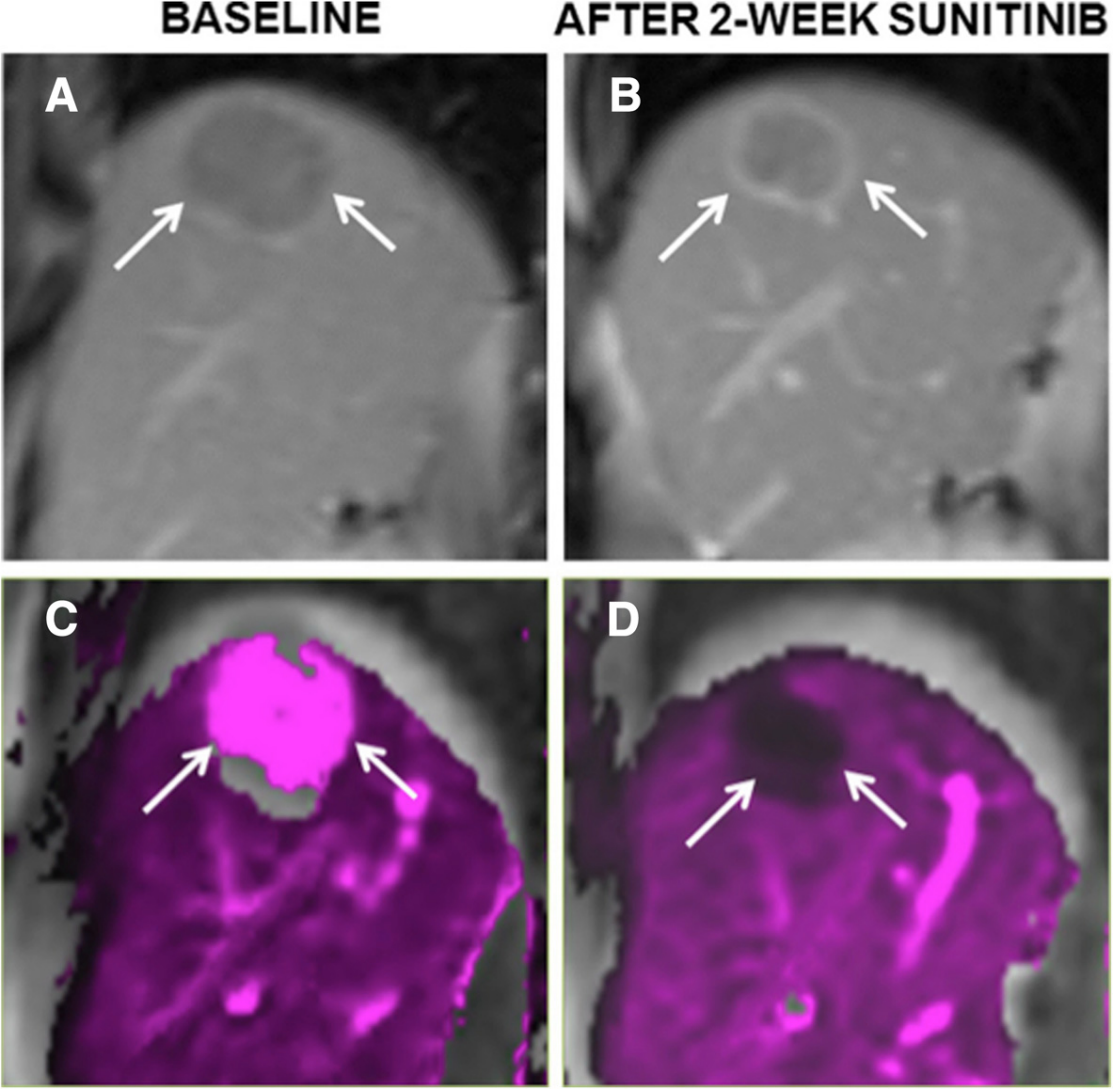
Functional transfer constant map of HCC during sunitinib therapy. Coronal enhanced MR T1-weighted images (A, B) and functional transfer constant (Ktrans) maps from MR perfusion (C, D) at baseline and after 2-week of sunitinib therapy in a 47-year-old man with HCC (arrows). After two weeks of treatment with sunitinib, HCC showed a 96% drop of Ktrans, which presented a conspicuity of permeability change.
